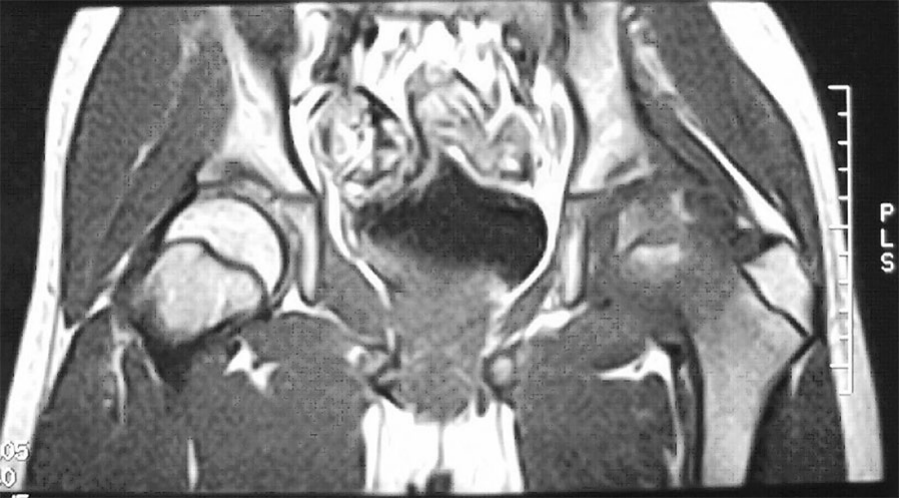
The coronal MRI of bilateral hip joints, the left acetabulum became shallow, the femoral head was shifted upward slightly
